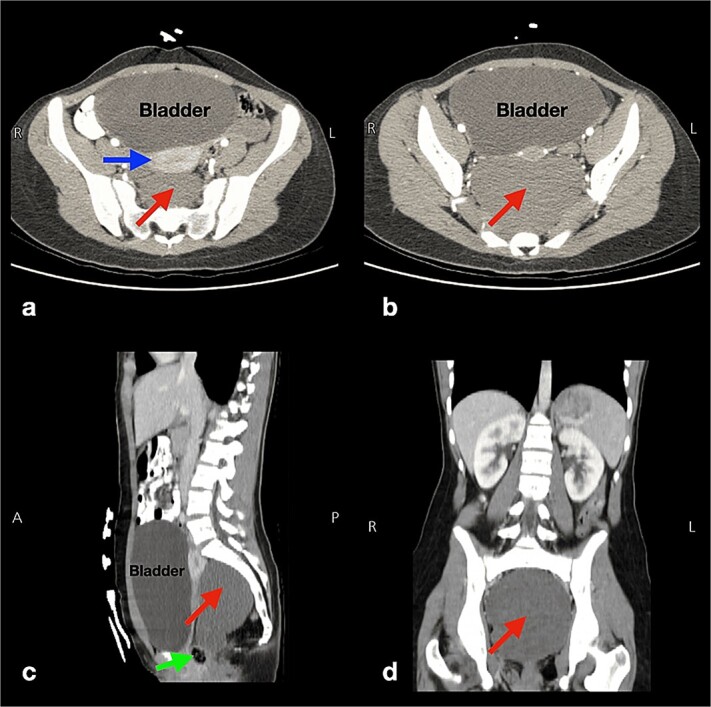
Multi-plane (a,b: axial and c: sagittal, d: coronal) abdominopelvic computed tomography scans displaying a well-defined cystic lesion, with fluid attenuation, and of biloculated nature measuring 11 × 10.5 × 8 cm (arrows in pictures a, b, c, d). This lesion has no soft tissue components or calcifications and is located in the presacral region near the sigmoid colon, rectum (arrow in picture c), and the uterus anteriorly (horizontal arrow in picture a), and both iliac vessels laterally. Also, the bladder is severely distended (pictures a, b, c).
